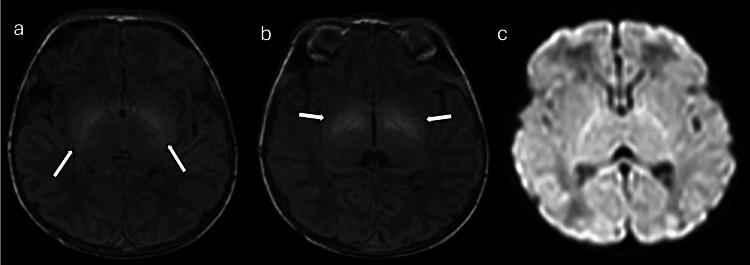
Kernicterus in a seven-day-old with hyperbilirubinemia (a) and (b) T1W MRI, showing a mild increase in T1 signal involving the bilateral globus pallidus and subthalamic nuclei (arrows), and (c) DWI with no evidence of restricted diffusion. Images obtained by the authors from the institutional PACS. All images are de-identified and used in accordance with institutional policy. T1W: T1-weighted; DWI: diffusion-weighted imaging; PACS: picture archiving and communication system
